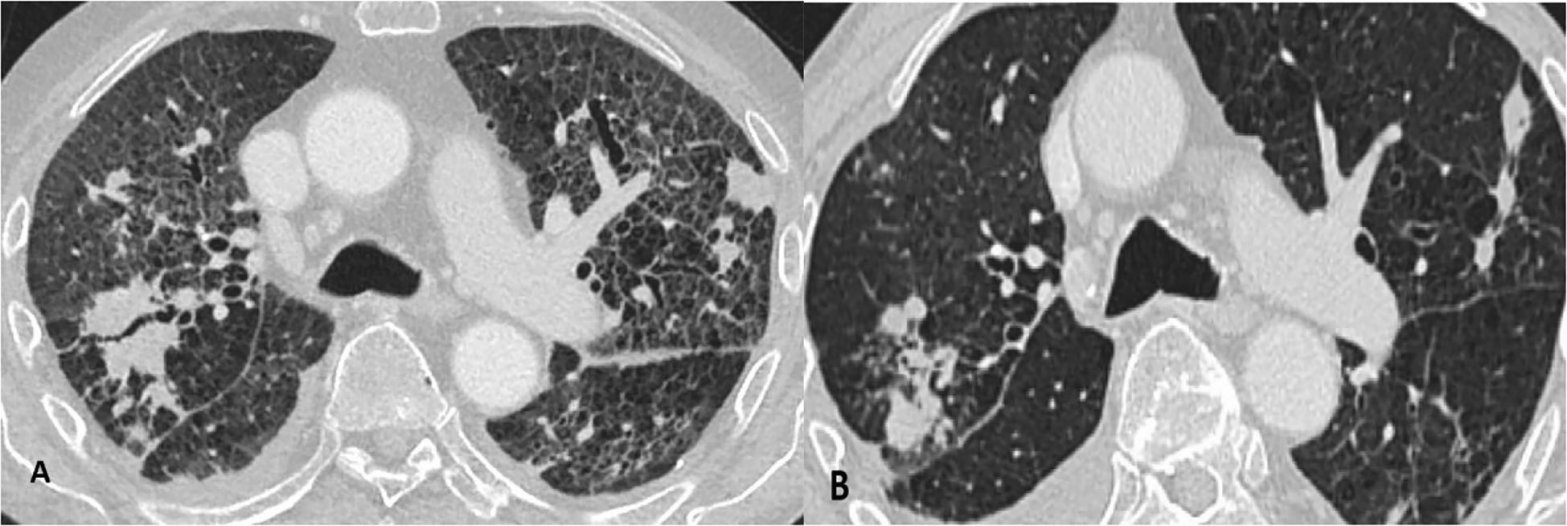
Axial reconstruction of chest HRCT before (a) and after (b) the treatment
